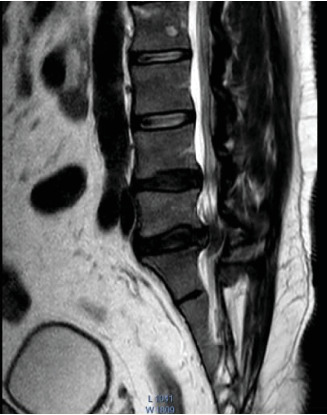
MRI showing posterior bulge at L5-S1 level causing severe spinal canal stenosis with compression of cauda equina nerve root.
